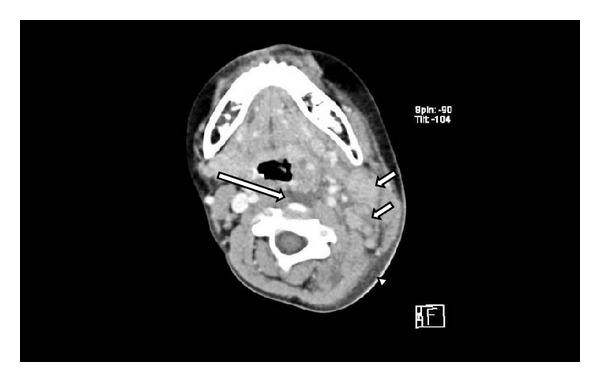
Postcontrast computed tomography of the neck showing left cervical lymph nodes swelling (arrow) with edema and increased density of adjacent subcutaneous tissue layer (arrowhead), and retropharyngeal low density without ring enhancement (long arrow).
